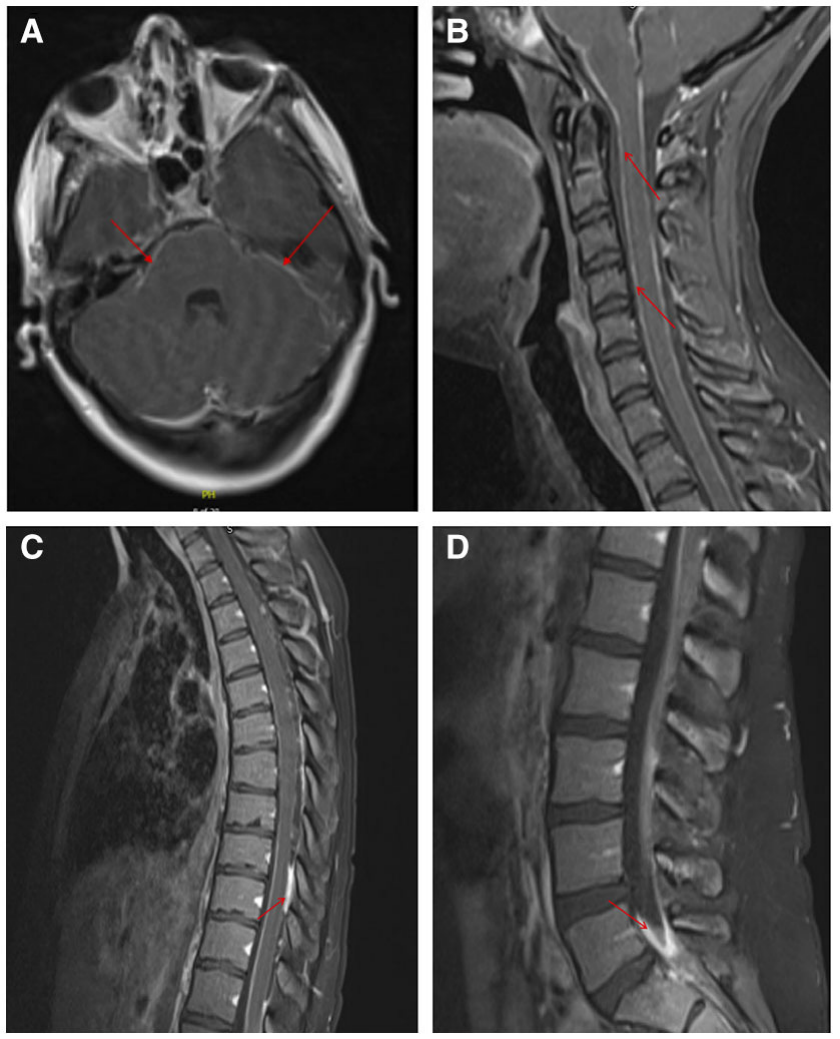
Leptomeningeal enhancement demonstrated on MRI with and without contrast of the brain and spinal cord. MRI brain axial image showing leptomeningeal enhancement of the posterior fossa and the visualized proximal spinal cord (A). Leptomeningeal enhancement was noted in sagittal images of the cervical spine (B), thoracic spine (C), and lumbar spine (D). Areas of hyperintensity are denoted by the red arrows.
